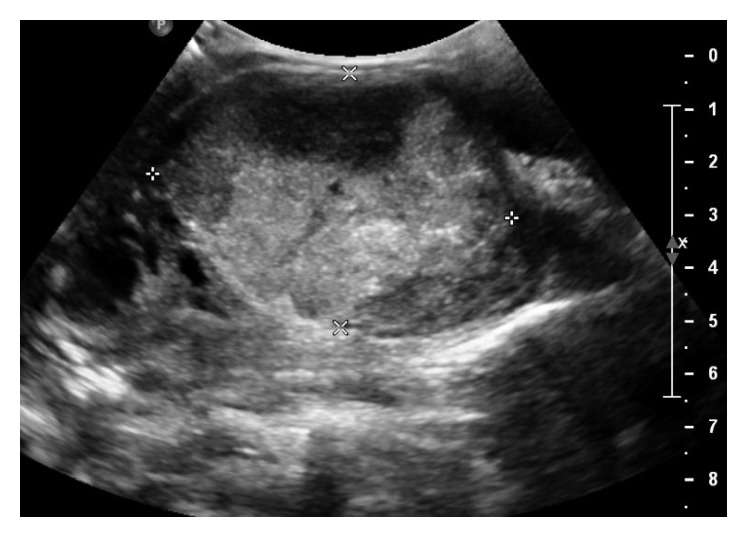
Thyroid ultrasound on hospital day 4 showed a 6.8 × 4.8 × 4.5 cm heterogeneous, necrotic, and hypervascular mass in a sagittal view of the right lobe.
